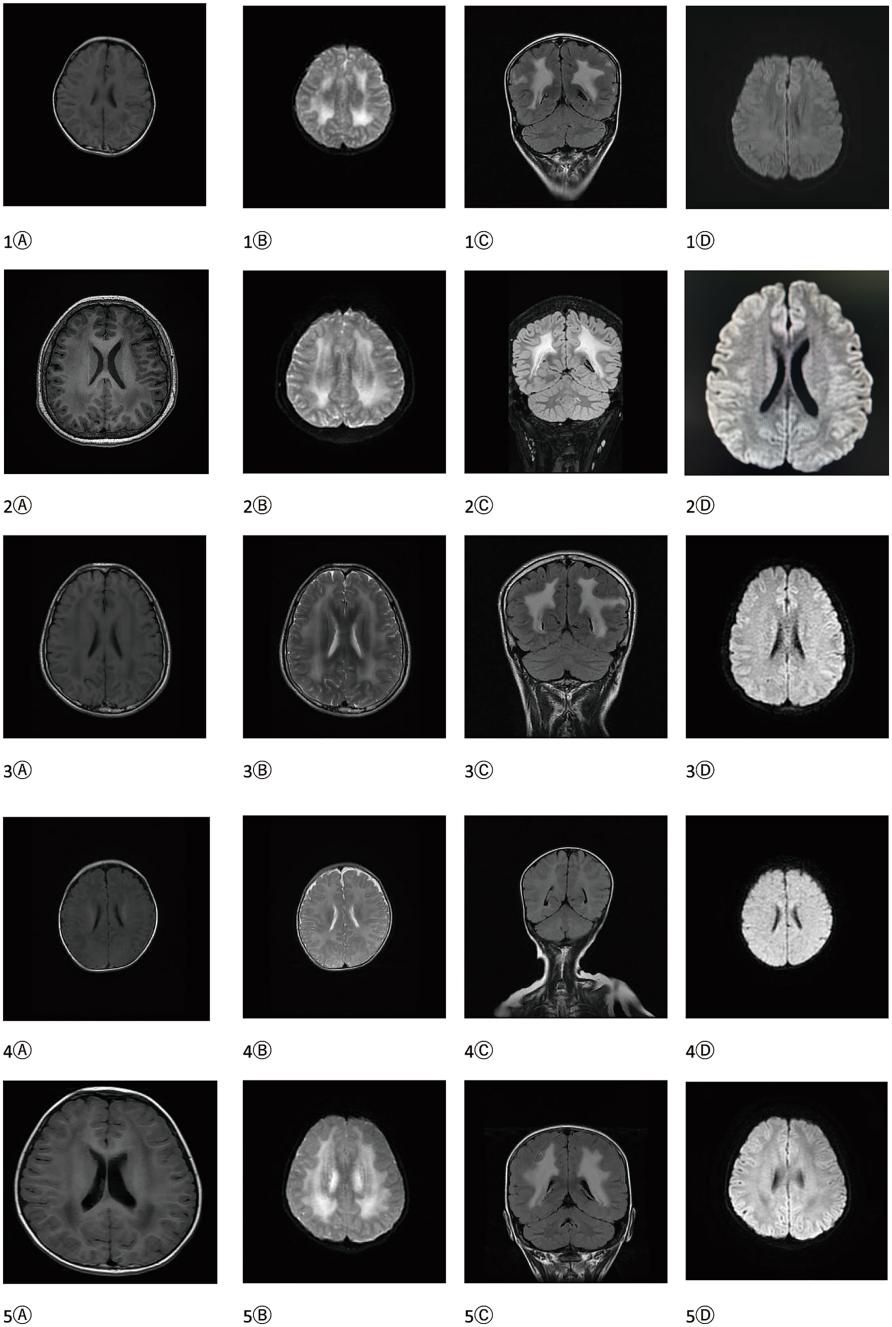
Cranial MRI of all the enrolled patients. Roman numerals indicate the case number, Ⓐ T1W sequence, Ⓑ T2W sequence, Ⓒ T2Flair sequence and Ⓓ DWI sequence.
